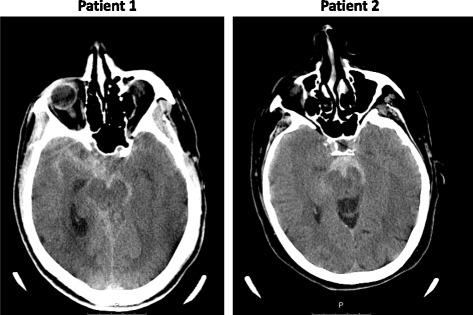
Pre-hospital cerebral CT scans from the MSU. PATIENT 1: Pre-hospital CT scan. There is subarachnoid hemorrhage located around the brainstem, in the supracellar cistern and in the right lateral fissure. PATIENT 2: Pre-hospital CT scan. There is subarachnoid hemorrhage mainly located ventral to the brainstem
